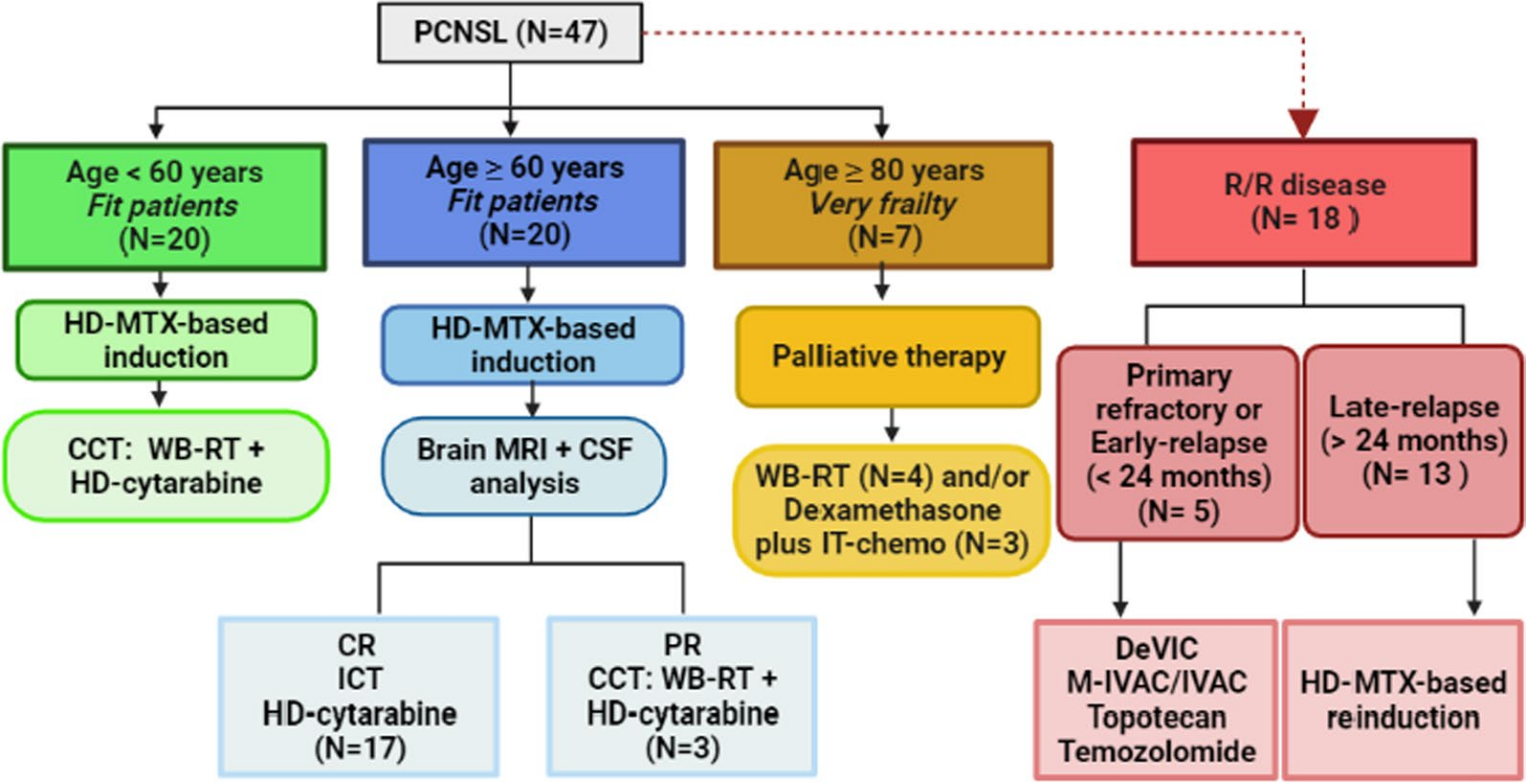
Therapeutic approach of the 47-Brazilian patients with PCNSL included in the study. *PCNSL* primary central nervous system lymphoma, *HD-MTX* high-dose methotrexate, *CCT* combined consolidation therapy, *HD-cytarabine* high-dose cytarabine, *MRI* magnetic resonance imaging, *CSF* cerebrospinal fluid, *CR* complete response, *PR* partial response, *WBRT* whole-brain radiotherapy, *IT* intratecal, *R/R* relapsed/refractory disease, *DeVIC* dexamethasone, etoposide, ifosfamide and carboplatin, *M-IVAC* methotrexate, ifosfamide, etoposide, cytarabine. *Among the 13 patients with late-relapse, only 5 cases received HD-MTX-based rescue therapy. The remaining 8 patients died before a new treatment
